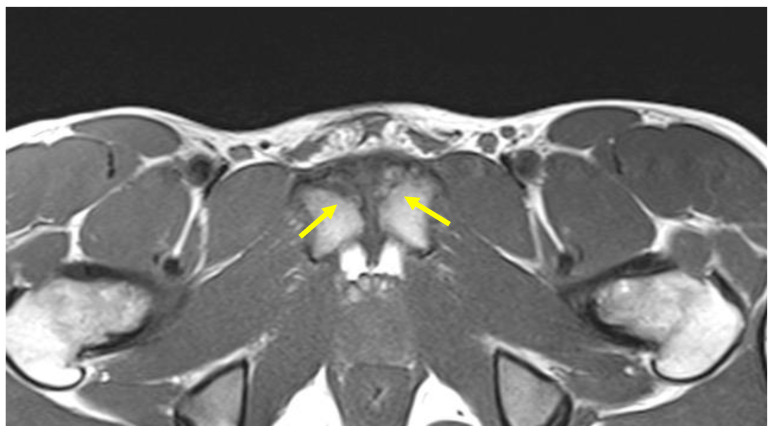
T1-weighted axial MR image of an eighteen-year-old patient showing two normal endochondral ossification nuclei (arrow).
